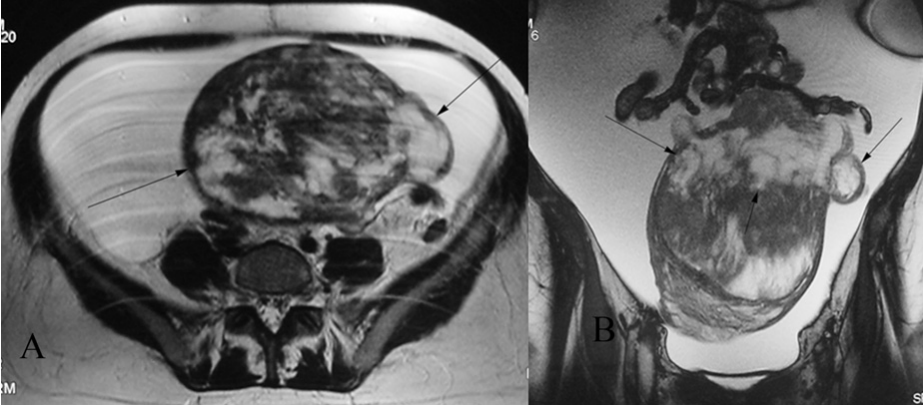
(A) Axial and (B) coronal T2-weighted fast spin echo magnetic resonance images which show a large ovoid subserosal leiomyoma in the pelvis that extends superiorly from the body and fundus of the uterus. The mass is heterogeneous with predominantly low to intermediate signal intensity relative to that of the outer myometrium. Several small foci of very high signal intensity are also seen (arrows). A normal endometrial stripe and junctional zone are seen. Note the presence of massive ascites.
